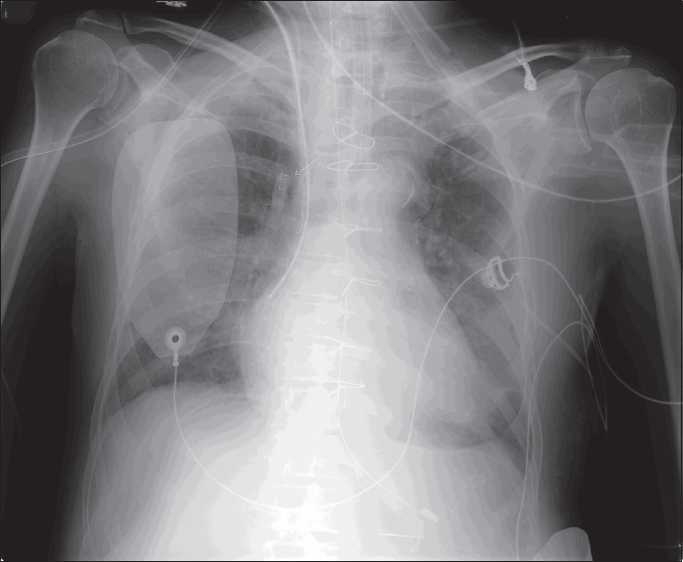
Chest radiograph showing metallic object lateral to the right sternal border
